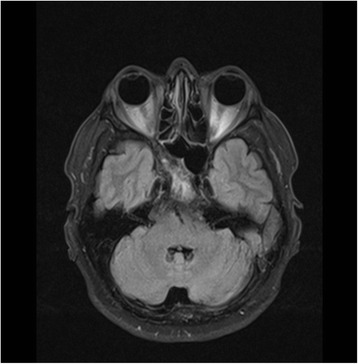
Brain MRI showing a tumor in the clivus and involvement of sphenoid bone
